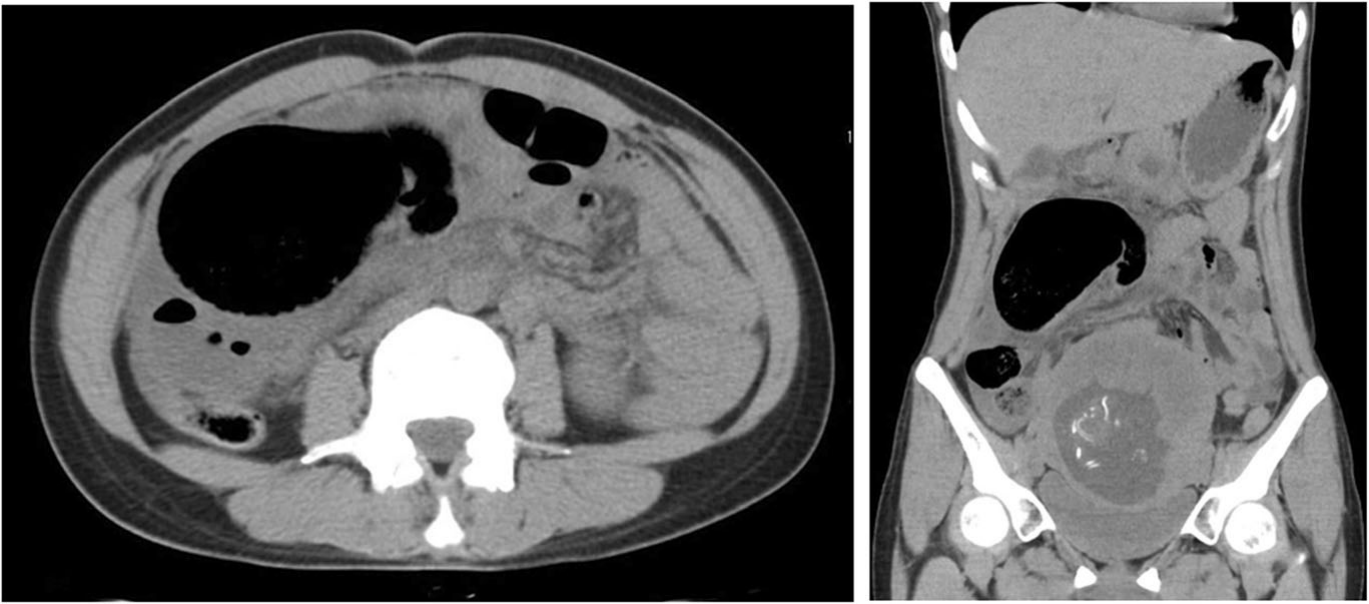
CT imaging of the abdomen (left, axial view; right, coronal view) In the right mid-abdomen, there was a large air containing cavity connected to the small intestine
